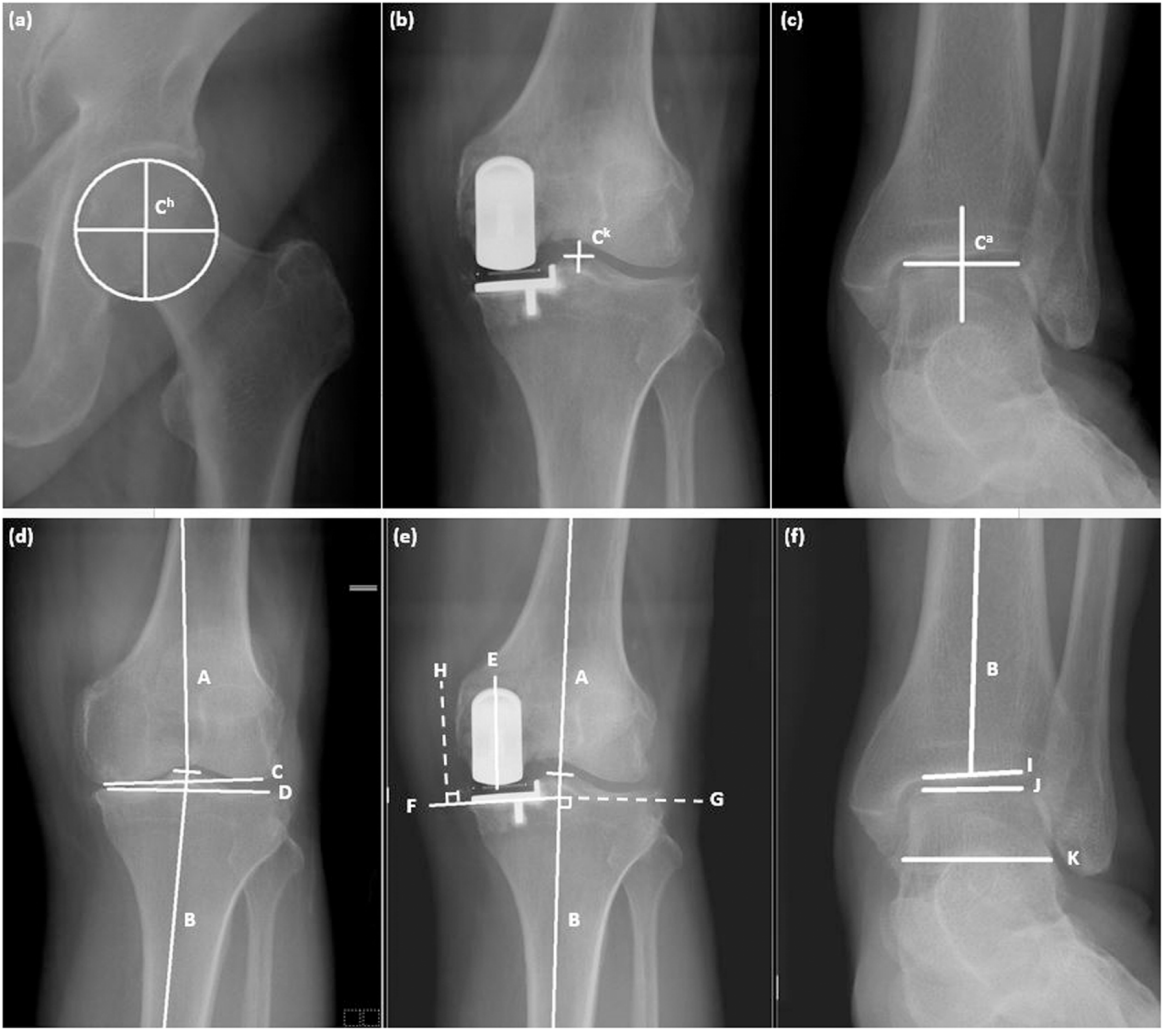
Radiographic measurements of knee and ankle joints. (a) The hip center (C^h^) is defined as the center of the femoral head. (b) The knee center (C^k^) is defined as the midpoint between the tibial spine tips. (c) The ankle center (C^a^) is defined as the midpoint of the articular surface of the talar dome. (d) The hip-knee-ankle angle (HKA) is defined as the angle between the mechanical axis of the femur (line A) and the mechanical axis of the tibia (line B). The lateral distal femoral angle (LDFA) is the lateral angle between line A and the joint line of the distal femur (line C). The medial proximal tibial angle (MPTA) is the medial angle between line B and the joint line of the proximal tibia (line D). The joint line convergence angle (JLCA) is the angle between line C and line D. (e) The femoral component alignment angle (FCA) is defined as the angle between line A and the longitudinal axis of the femoral component (line E). The tibial component alignment angle (TCA) is the angle between the undersurface of the tibial component (line F) and the vertical line to the mechanical axis of the tibia (line G). The component vergence angle (CVA) is the angle between line E and the vertical line to the undersurface of the tibial component (line H). (f) The tibial plafond-ground angle (PGA) is defined as the angle between the joint line of the tibial plafond (line I) and the ground line (line K). The talus-ground angle (TGA) is the angle between the joint line of the talar dome (line J) and line K. The tibial plafond-talus angle (PTA) is the angle between line I and line J.
